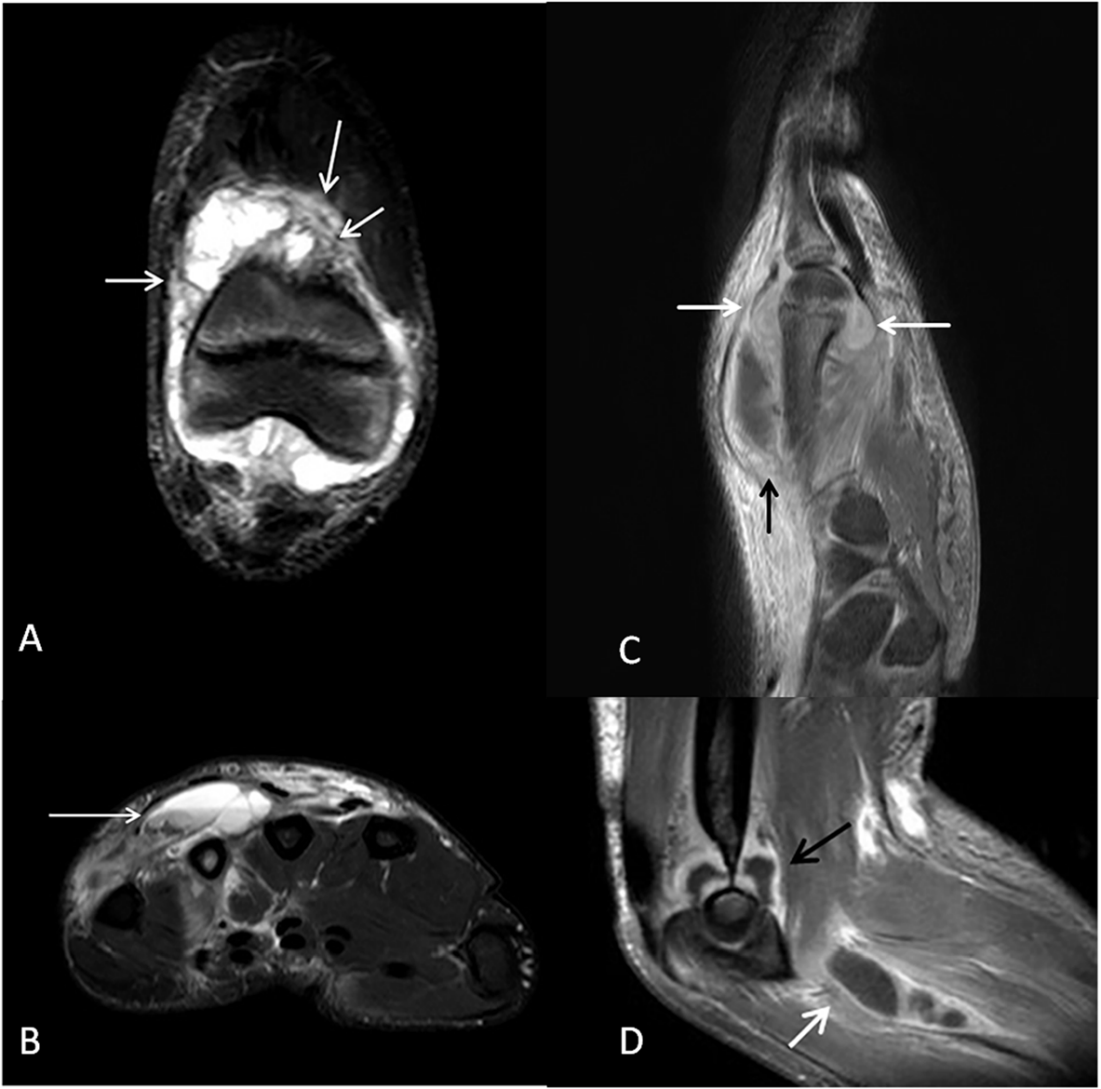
Different presentations of osteoarticular inflammatory involvement in a girl with pyogenic sterile arthritis, pyoderma gangrenosum and acne (PAPA) syndrome. a Coronal STIR of the anterior right knee demonstrates moderate effusion with synovial thickening (arrows) and surrounding soft tissue edema. b Axial STIR image at the mid-level of the metacarpals of the left hand demonstrates abnormal bone marrow signal of the fourth metacarpal bone with an associated loculated dorsal fluid collection that demonstrates a fluid-fluid level (arrow). c Sagittal T1-weighted image with fat suppression and intravenous contrast administration through the fourth metacarpal bone at the same presentation demonstrates ring enhancement of the dorsal fluid collection (black arrow). Moreover, there is extensive synovial thickening and enhancement of the fourth metacarpophalangeal joint (white arrows). d Sagittal T1-weigthed image of the right elbow with fat suppression and after intravenous gadolinium administration demonstrates moderate effusion with synovial thickening and enhancement (black arrow). There is a ring-enhancing fluid collection (abscess) extending from the joint into the soft tissue antero-medially (white arrow)
